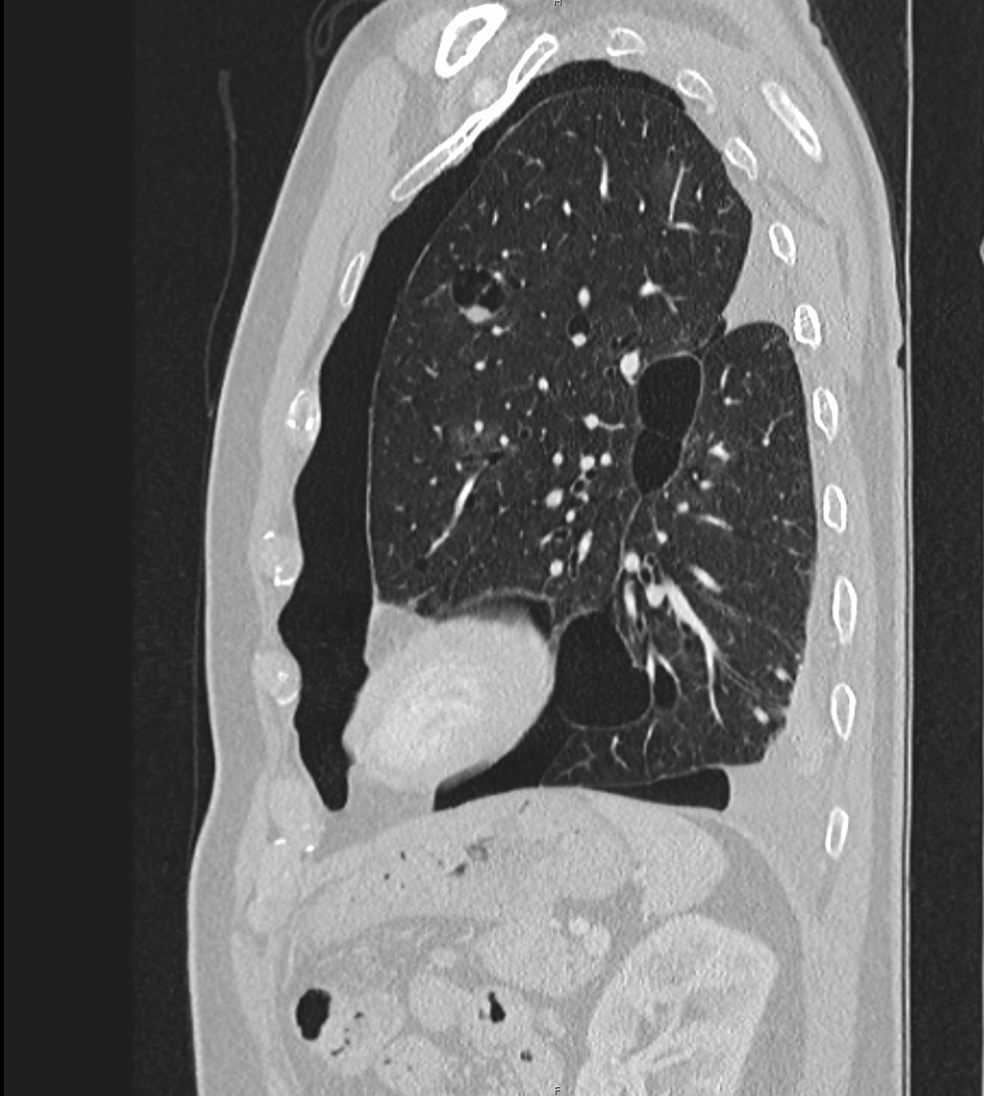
Computed tomography (CT) scan of left lung revealing hydropneumothorax; this was from nine months prior to the current visit when the patient presented with four months of persistent cough, shortness of breath, and a cold
